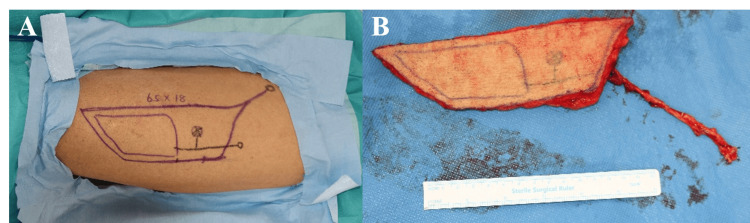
ALT (anterolateral thigh) flap design and harvest (A) Preoperative skin markings for a 6.5 × 18 cm ALT flap on the left thigh. (B) Harvested flap with a musculocutaneous perforator identified preoperatively by duplex ultrasonography, arising from the descending branch of the lateral circumflex femoral artery. The ALT is a commonly used soft-tissue flap for tongue reconstruction due to its reliable anatomy and adequate bulk.
